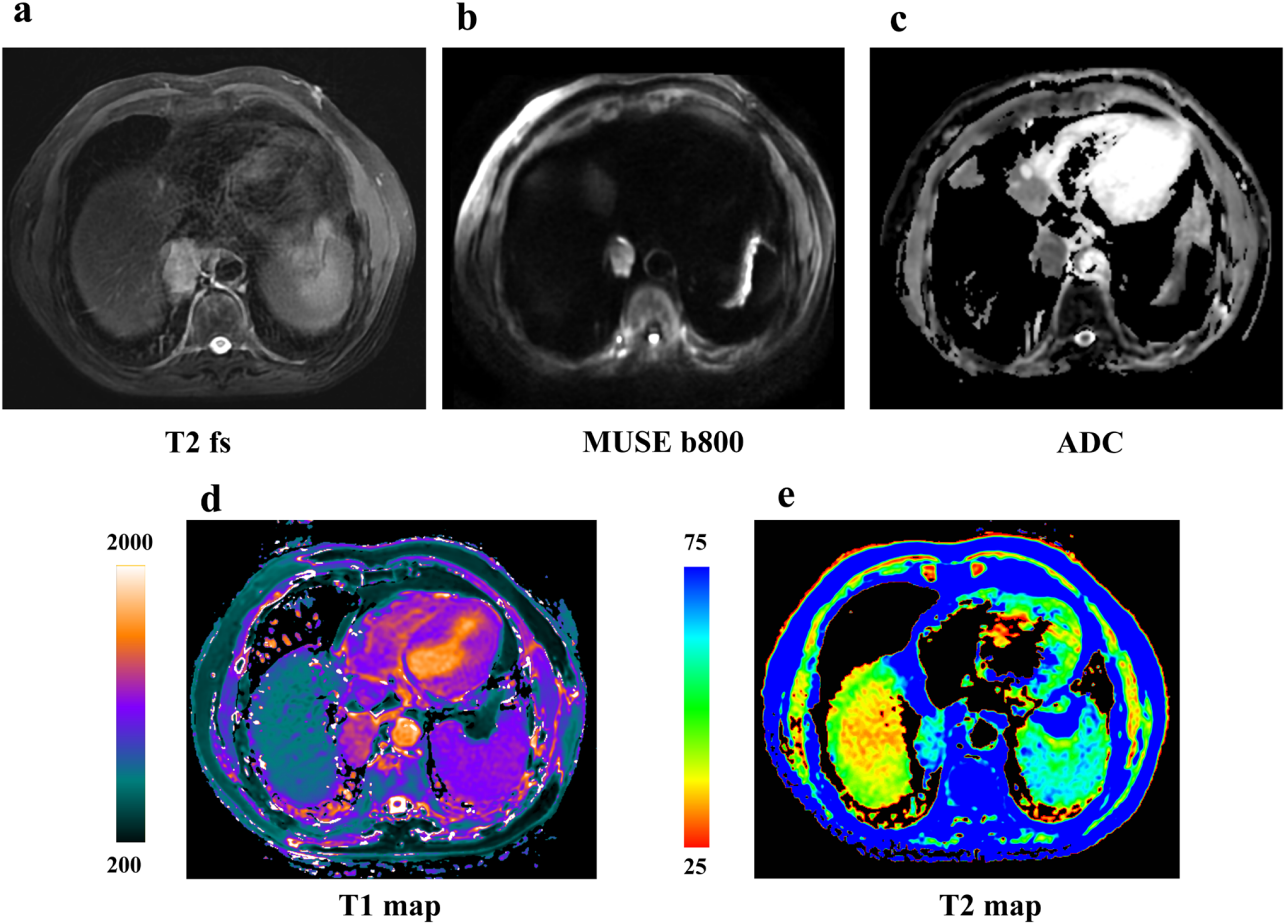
Male, 71 years old, soft tissue mass in the lower lobe of the right lung, about 43 mm in size, pathologically confirmed as squamous carcinoma. (a) T2WI; (b) MUSE DWI (*b* = 800 s/mm^2^); (c) MUSE ADC; (d) T1 mapping (ms); and (e) T2 mapping (ms).
